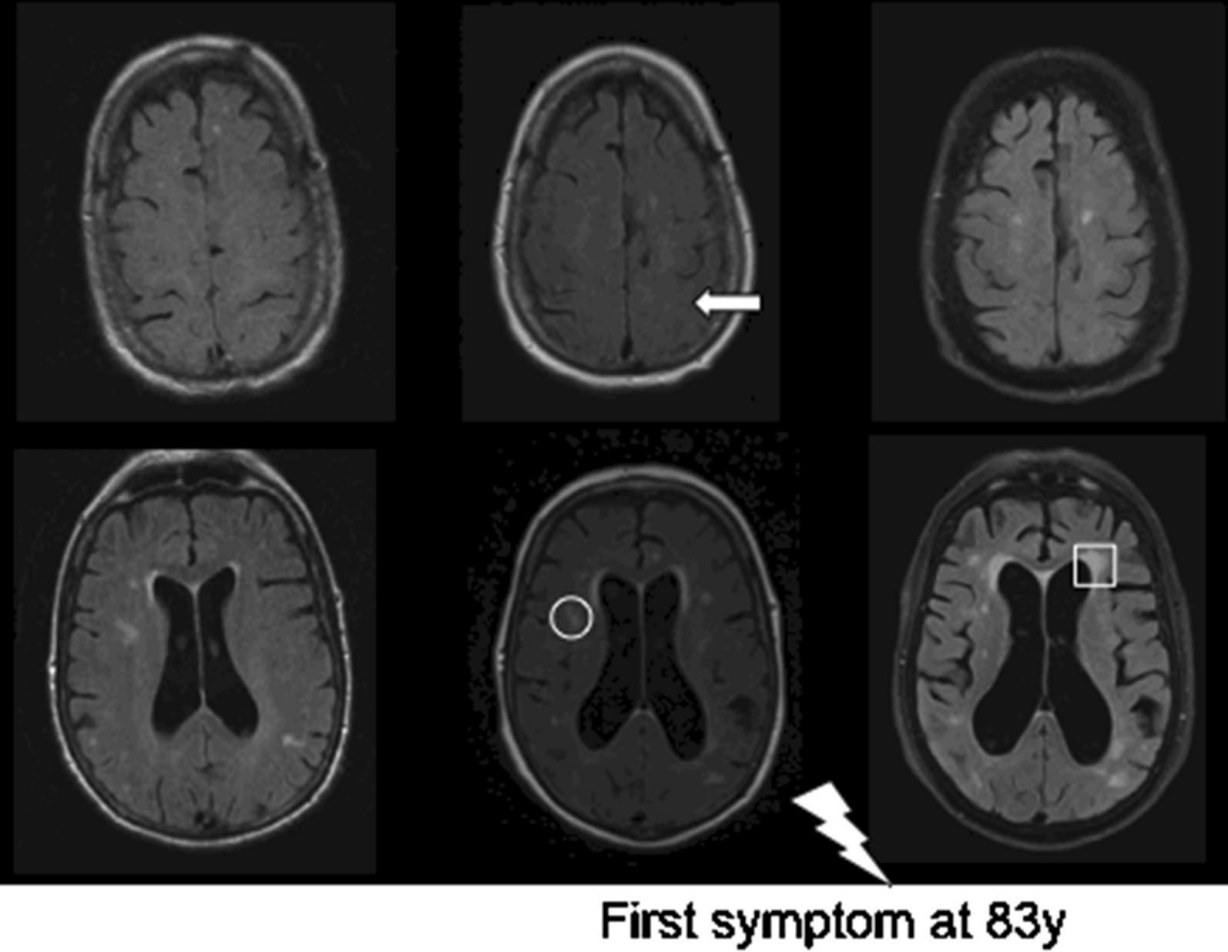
Pre- and post-symptomatic magnetic resonance images of a patient that developed symptoms after 10 years. The MRI images show the changes in ventricle width, deterioration of white matter hyperintensities (circle: deep; square: periventricular) and tight high convexity (arrow) all before onset of symptoms (thunderbolt) of a typical case. Left: 10 years before symptom onset. Middle: 4 years before symptom onset. Right: 6 months after symptom onset
